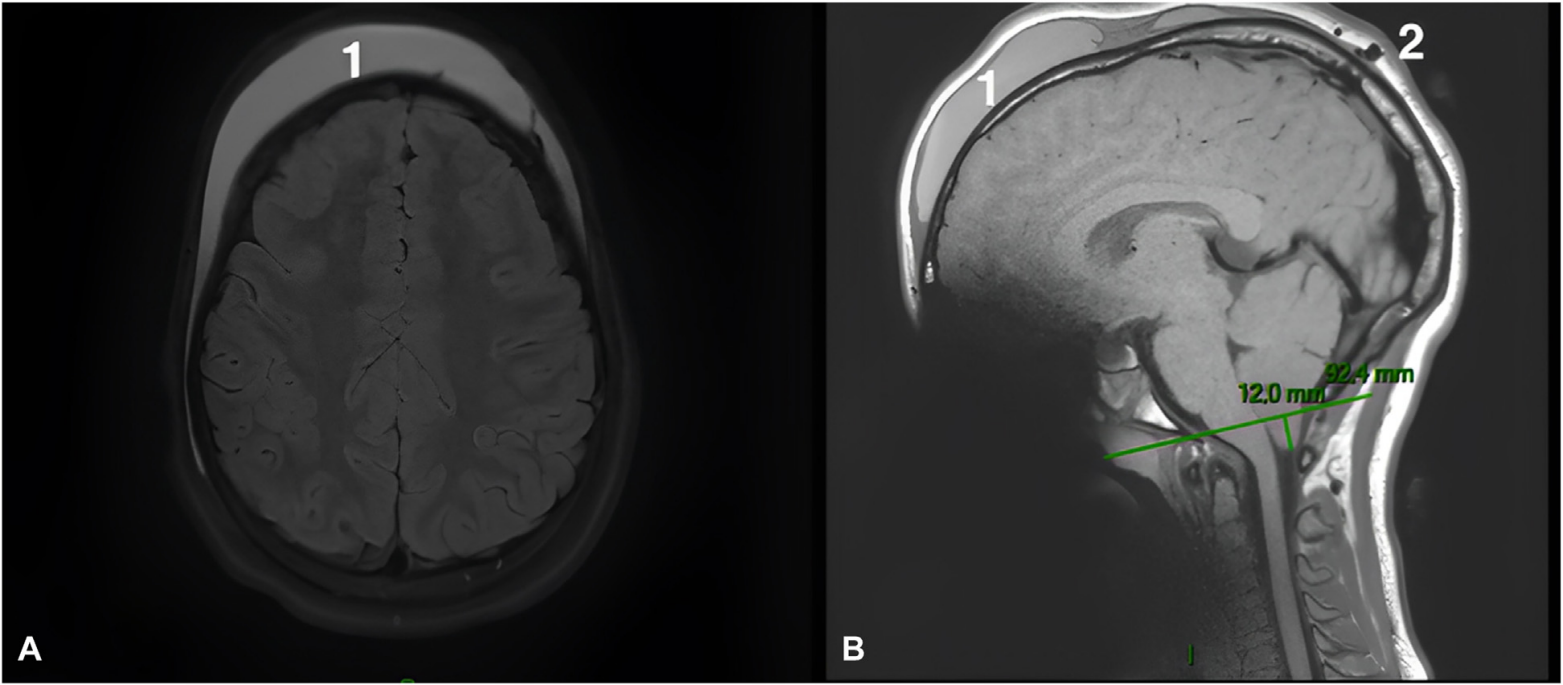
A, Magnetic resonance imaging frontal section T2 fat sat sequence showing this large subgaleal collection (1). B, Magnetic resonance imaging sagittal section T2 flair sequence showing the subgaleal collection (1) and the sinus pericranii in communication with the sagittal sinus (2).
